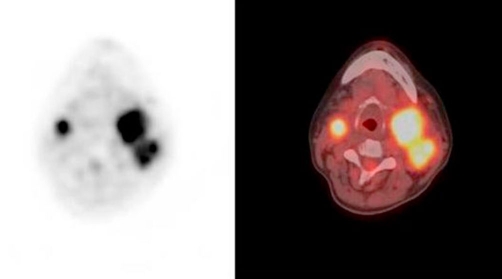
FDG-Positron Emission Tomography (PET) CT scans showed a mass at the left side (SUVmax: 9.9), and mass at the right side of the neck (SUVmax: 8.5).
